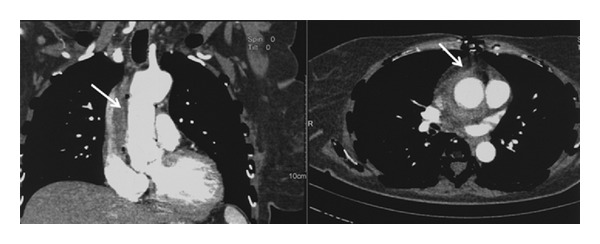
Thoracic CT scan with mediastinitis in regression.
